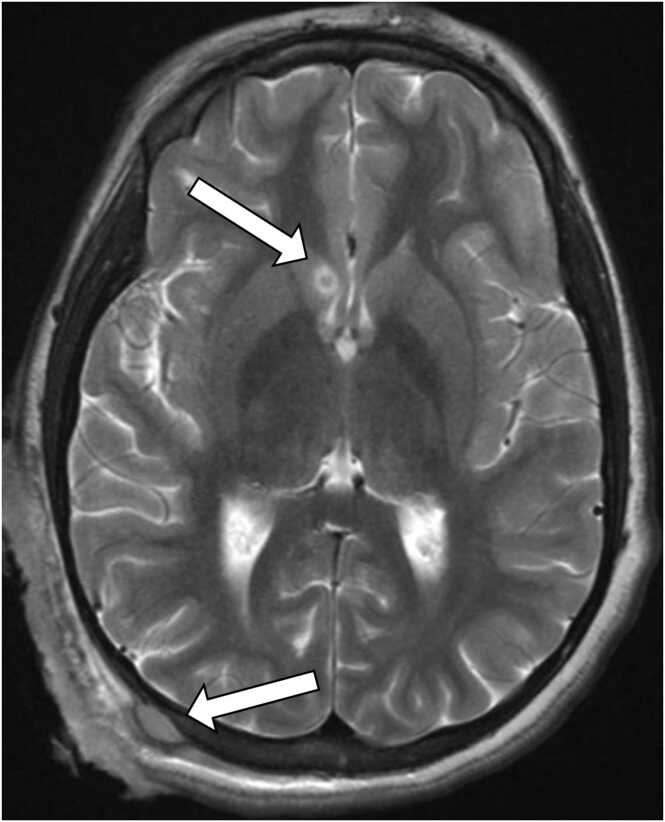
Axial brain MRI (T2) with findings of ulcerative, necrotic mass-like enhancement eroding the right occipital calvaria with epidural extension (bottom arrow); enhancing 4-mm focus around right caudate nucleus (top arrow).
